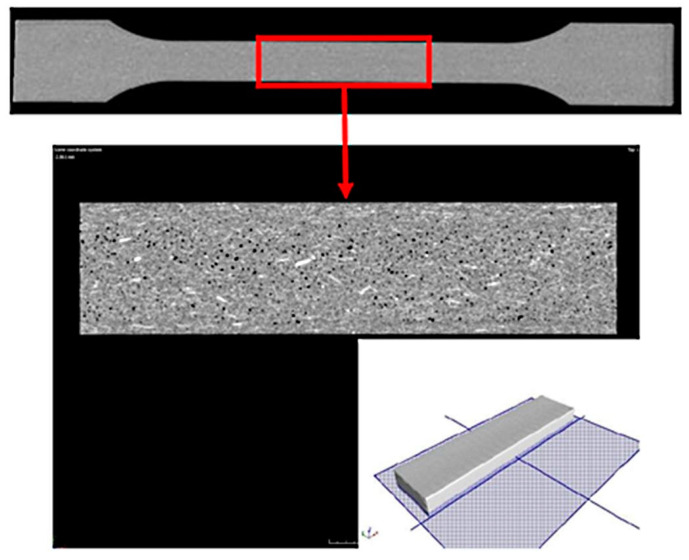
Sample test result using computed tomography with a resolution of 170 µm and a chosen area of the test sample made with a resolution of 50 µm with imaging of the test plane on the cross-section.
